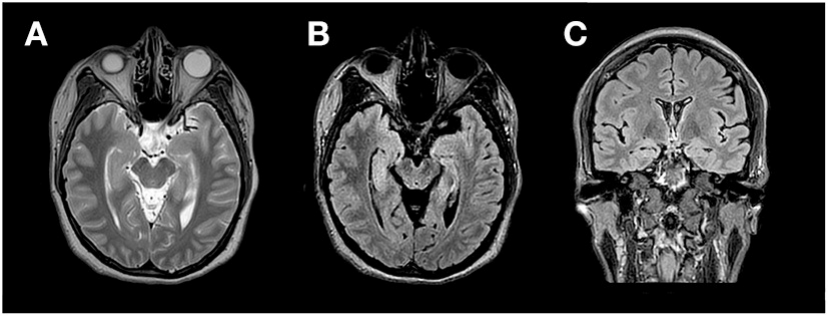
MRI (3.0T) at the time of presentation. Axial T2 Weighted Image (A), Axial Fluid attenuated inversion recovery (FLAIR) sequence (B) and coronal FLAIR (C) show cortical thickening and hyper intense signal in the medial aspect of bilateral temporal lobes Right > Left.
